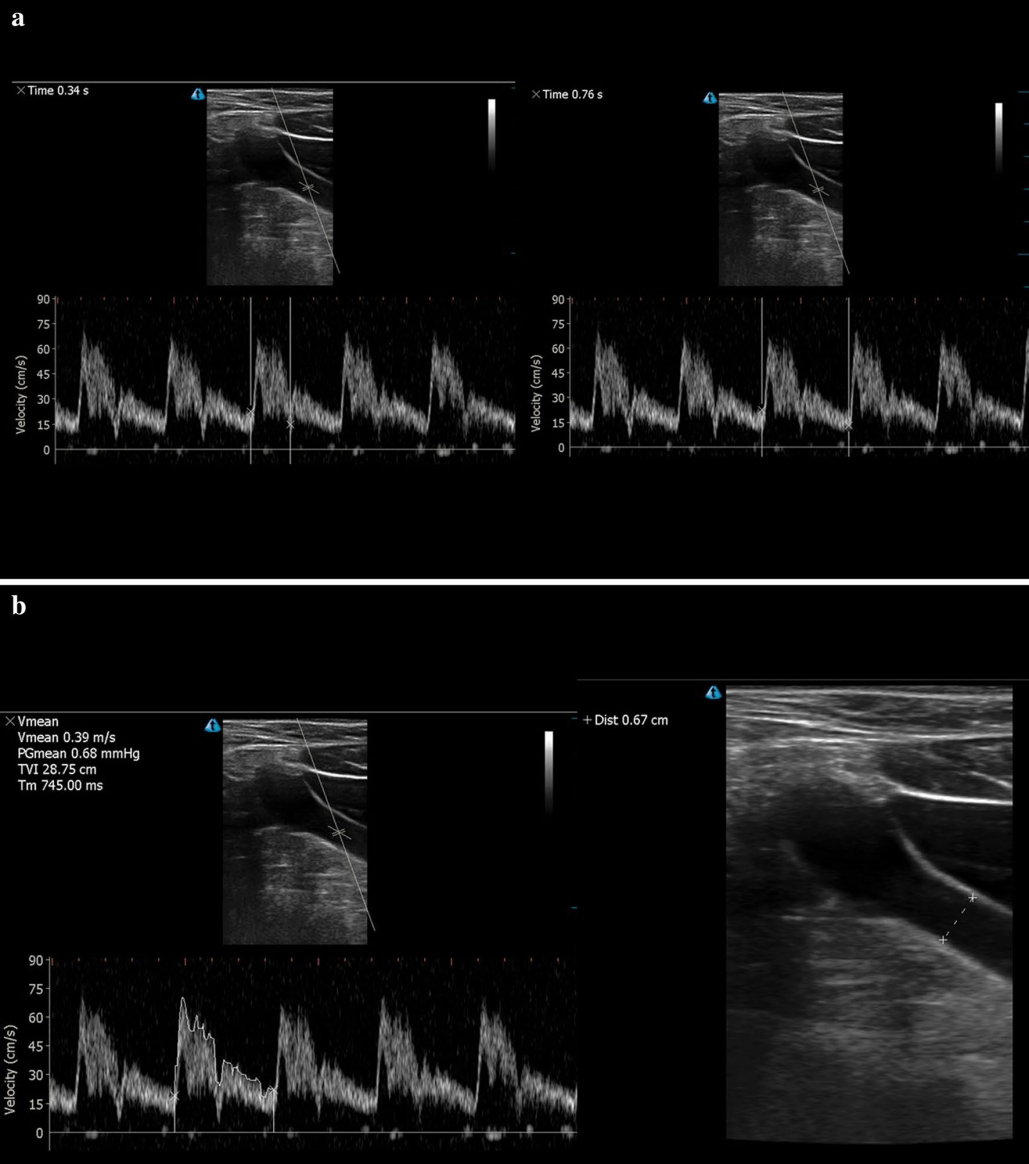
a Carotid systole time, as measured from the start of the systolic upstroke to the start of the dicrotic notch (*left*). Carotid cycle time, as measured from start of one systolic upstroke to the next (*right*). b Velocity time integral tracing of the spectral Doppler signal (*left*). Carotid diameter (*right*)
